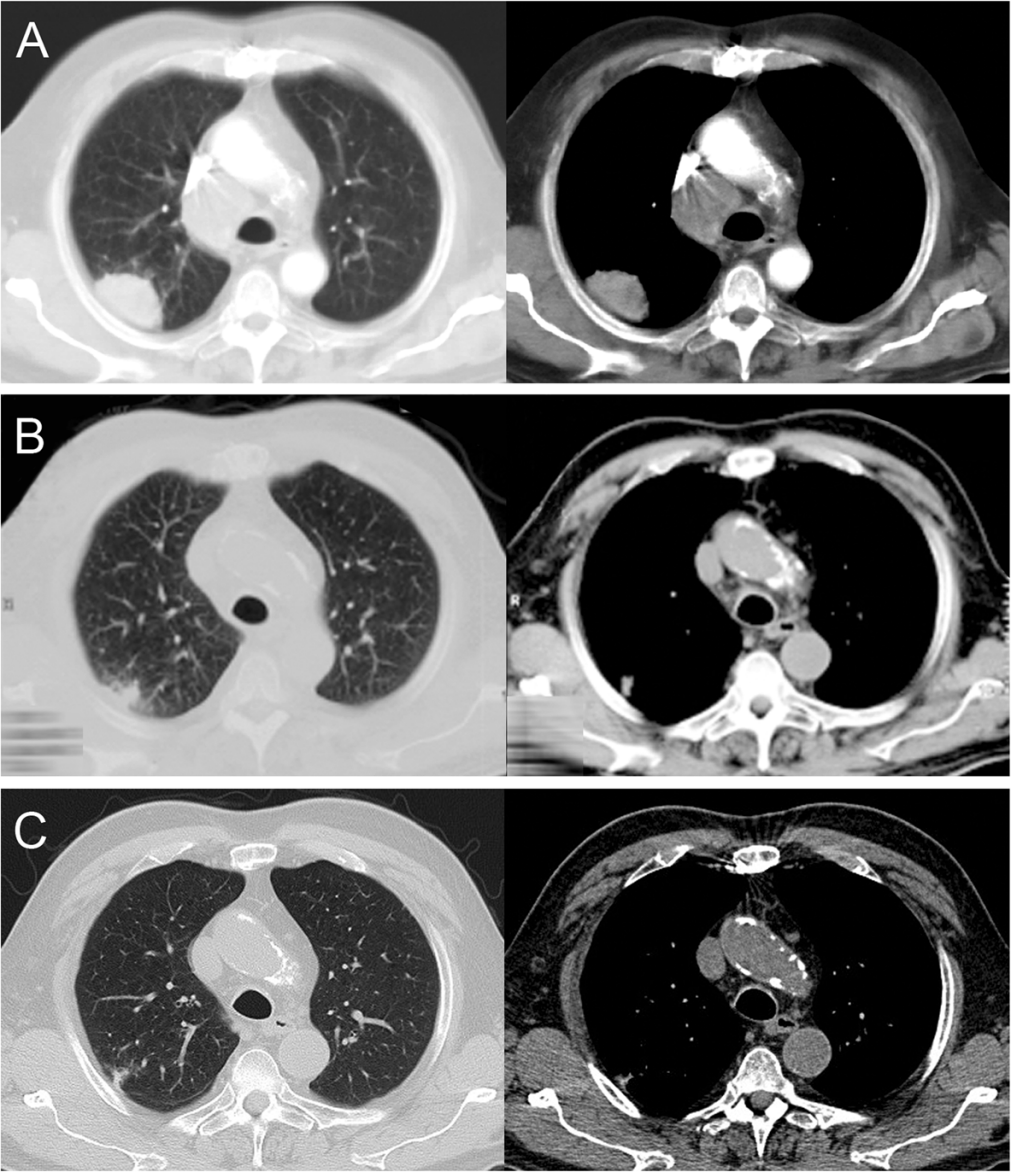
a Initial chest computed tomography scan showing the tumor in the upper lobe of the right lung and enlarged mediastinal lymph nodes in the paratracheal position. b Computed tomography scan after 2 months, c after 1 year
